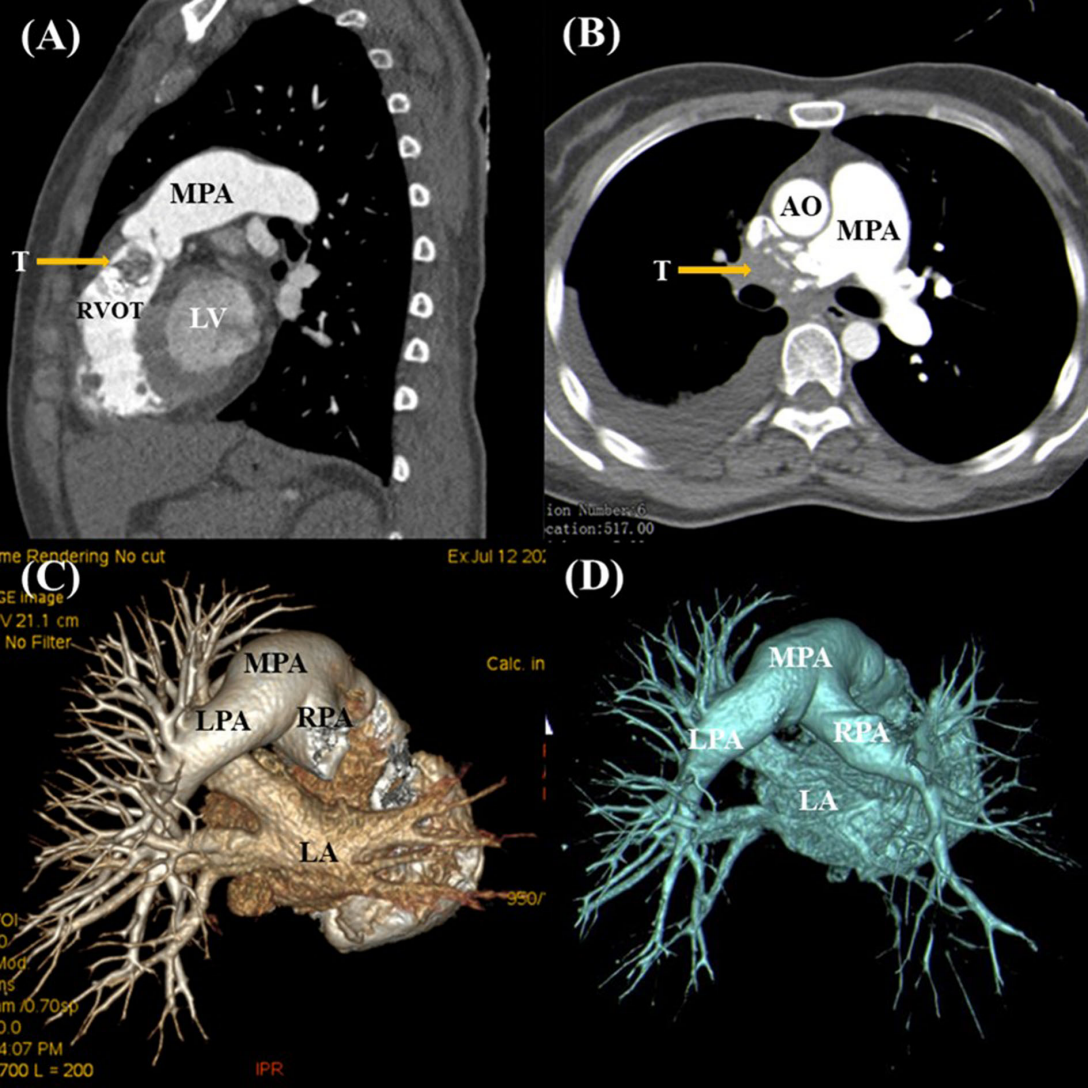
Cardiac computed tomography demonstrated a mixed-density lesion with irregular margins in the RVOT (A), along with another mass in the RPA that was completely occluded (B, C). Postoperative CT imaging showing re-establishment of RPA blood flow (D).
